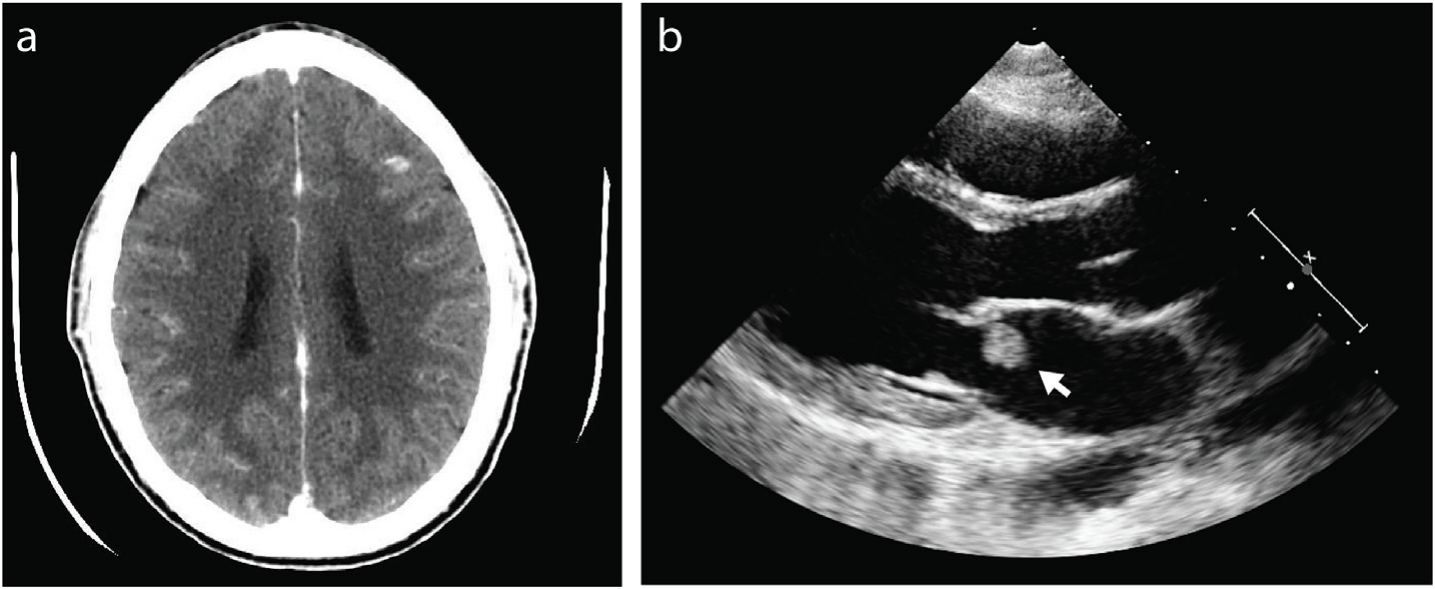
Computed tomography (CT) imaging of the brain showing multiple areas of abscesses (a) and echocardiography revealing mitral valve endocarditis (b, arrow).
